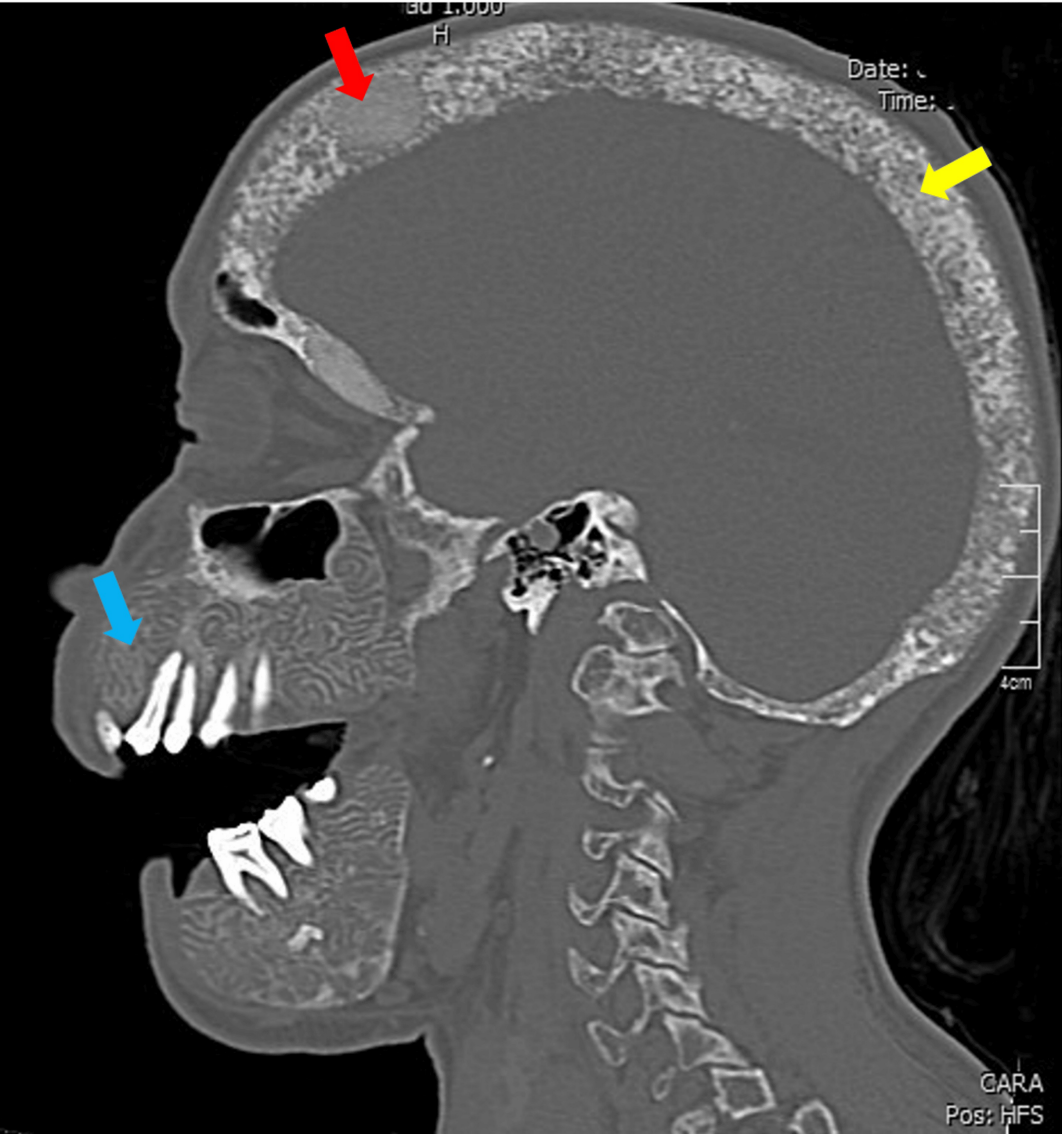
CT of the skull in bone window. Sagittal reconstruction showing diffuse craniofacial bone thickening and deformity, with marked maxillary and mandibular overgrowth with significant cortical expansion (blue arrow), diffuse “salt and pepper” appearance predominantly in the calvaria and skull base (yellow arrow), and rounded focal ground-glass regions within the calvarium (red arrow).
